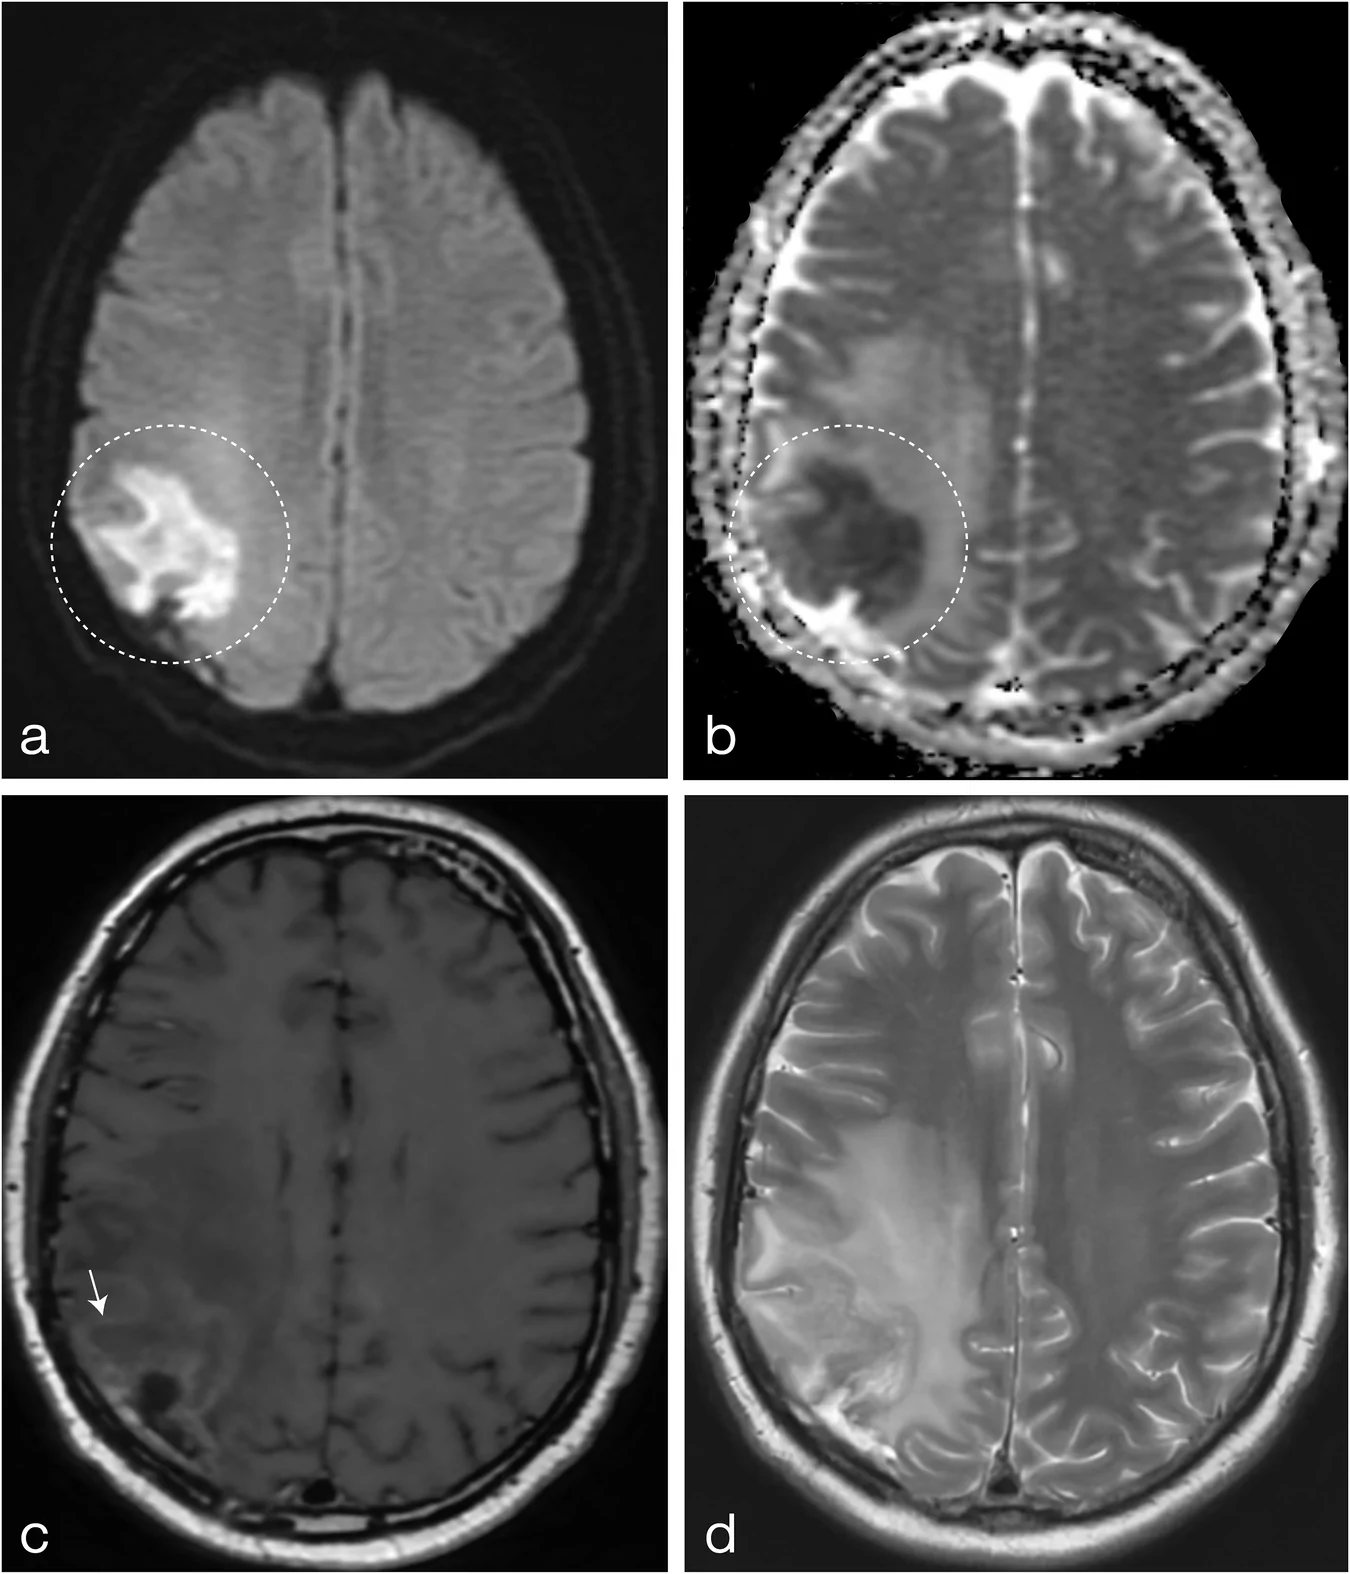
Bevacizumab-induced coagulative necrosis. A patient with a history of right-sided glioblastoma after surgical debulking, chemoradiotherapy and adjuvant temozolomide, with residual/recurrent disease; the patient is on bevacizumab. a, b Axial diffusion-weighted image (DWI) and apparent diffusion coefficient (ADC) map showing geographic focal markedly restricted diffusion abutting the anterior aspect of the resection cavity (white dashed circles). c Axial post-contrast T1-weighted image at the same level; the white arrow indicates the area corresponding to the diffusion abnormality, which shows no enhancement; note the peripheral thin intrinsic high T1 signal. d Axial T2-weighted image at the same level demonstrating corresponding heterogeneous T2 hyperintensity
